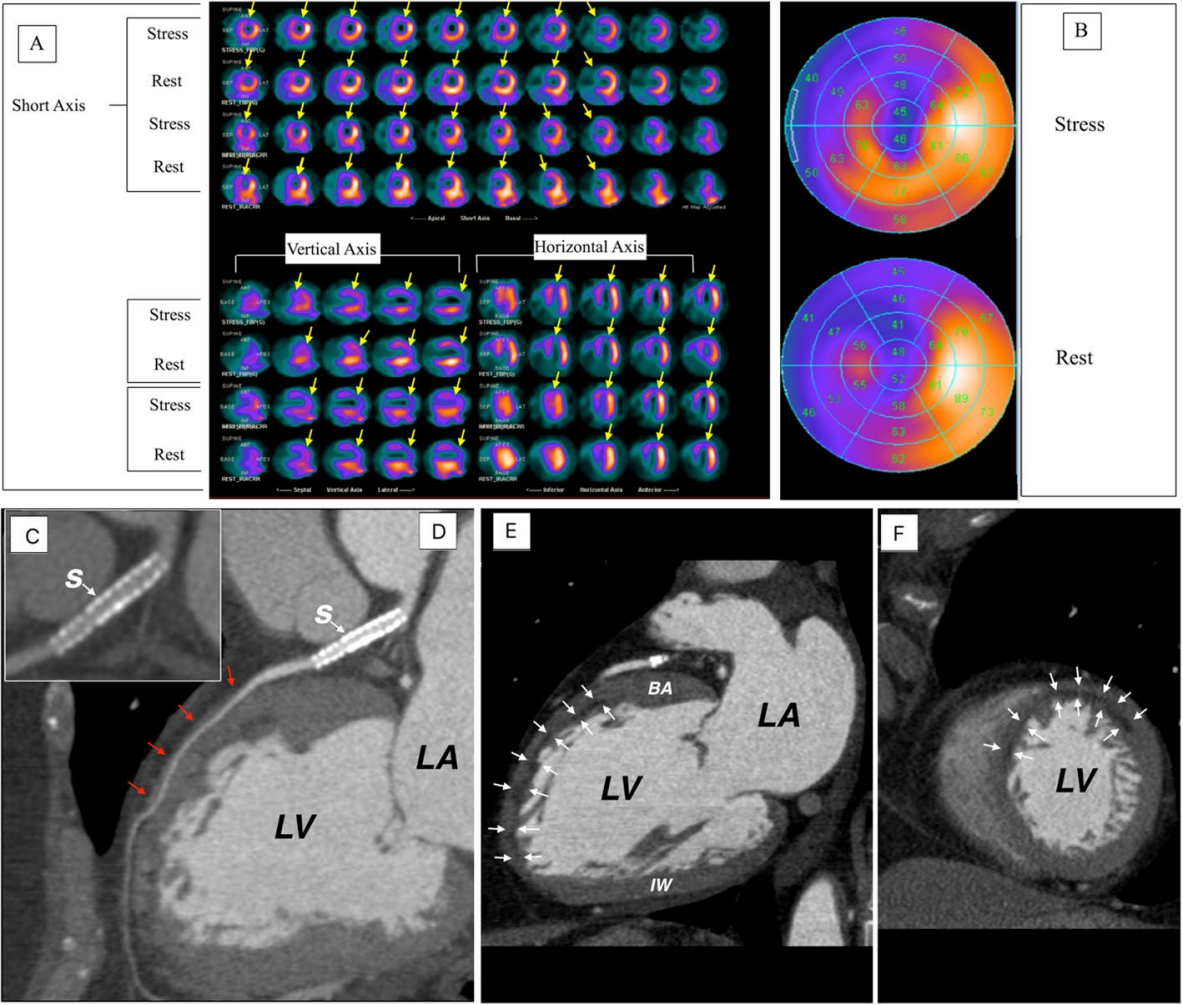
A SPECT images, B polar maps at stress (upper panel) and rest (lower panel), C–F computed tomographic angiography images. LV, left ventricle; LA, left atrium; BA, basal anterior wall; IW, inferior wall
